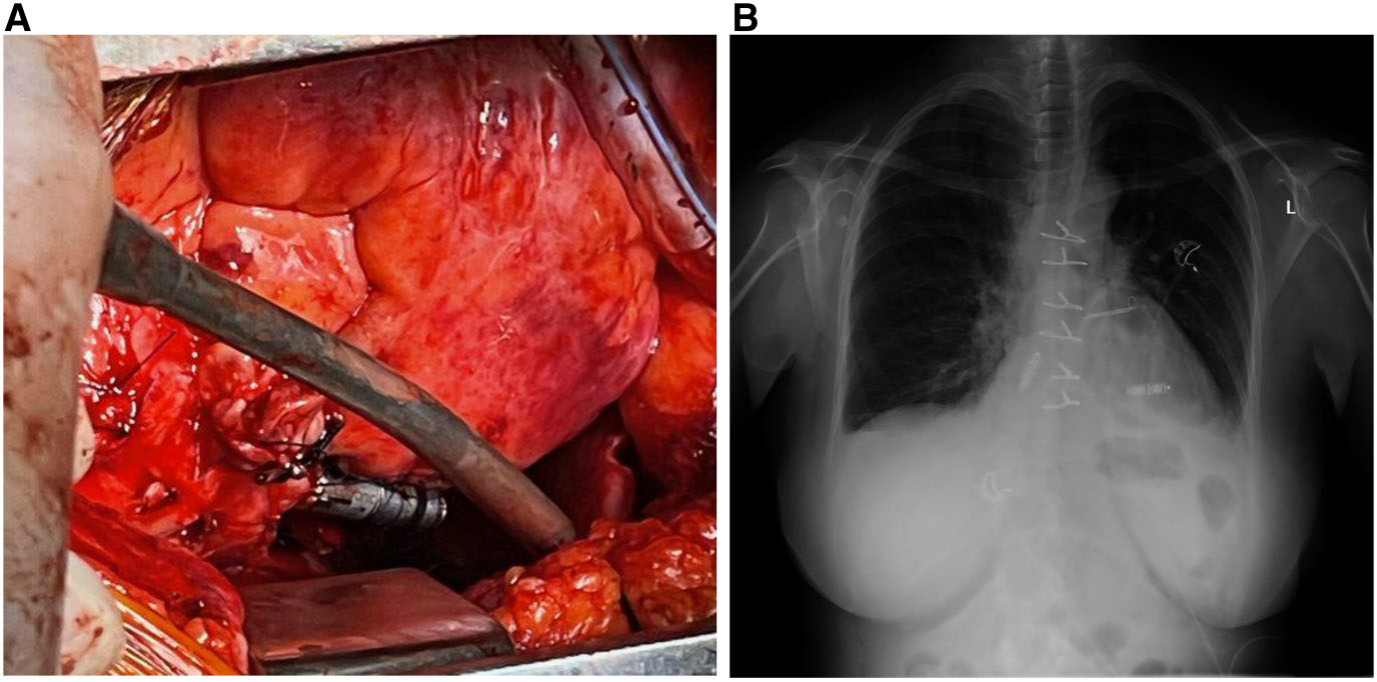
(A) Intraoperative photo of epicardial leadless pacemaker implantation. (B) Chest X-ray during follow-up visit.
